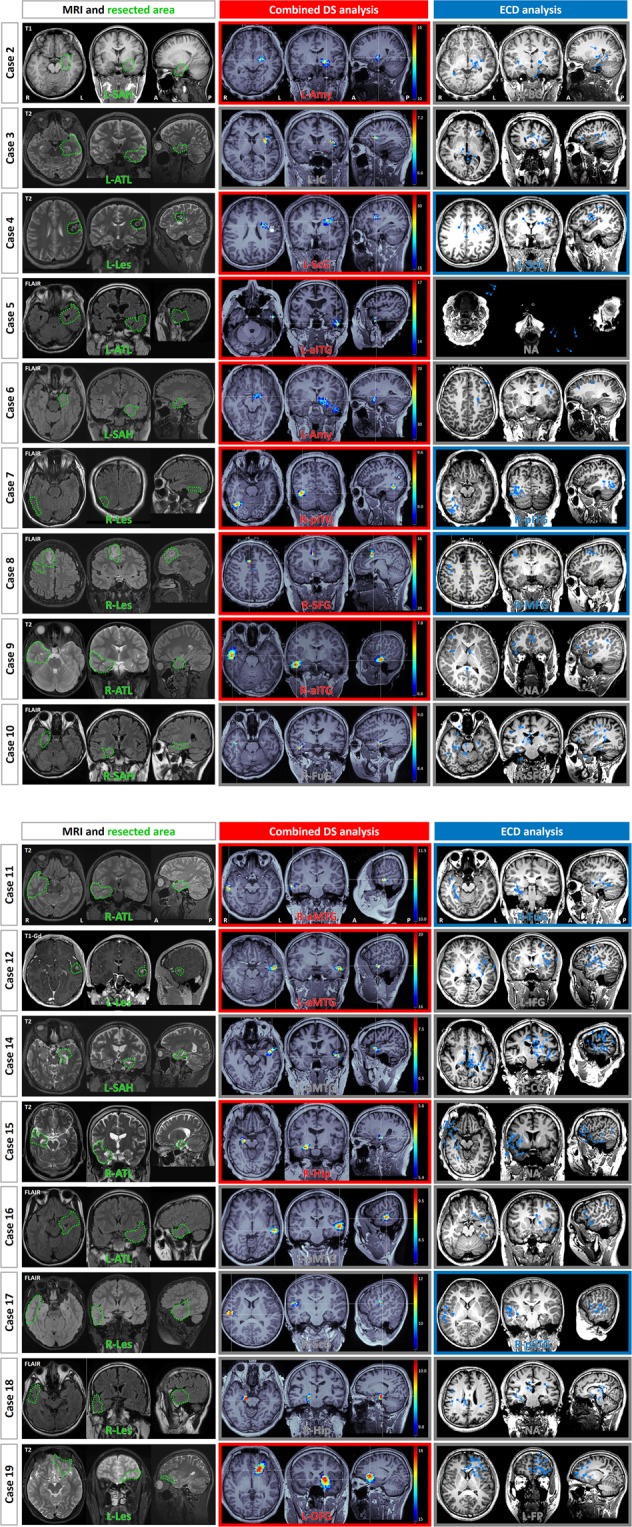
Resected area, cDS analysis, and ECD analysis of 16 cases. Left column: The green dotted line on the preoperative MRI represents the resected area and the surgical method are shown. Middle and right columns: Results of the cDS and ECD analyses showing the diagnosed focus area. When the estimated focus of cDS and ECD analyses was concordant with the resected area, the focus was described by colored letters and a colored frame. When they were discordant, the letters and frame were gray. Abbreviations: cDS, combined distributed source; ECD, equivalent current dipole; SAH, selective amygdalohippocampectomy; ATL, anterior temporal lobectomy; Les, lesionectomy; CC, corpus callosum; Hip, hippocampus; Amy, amygdala; FuG, fusiform gyrus; a-, anterior; p-, posterior; STG, superior temporal gyrus; MTG, middle temporal gyrus; ITG, inferior temporal gyrus; FP, frontal pole; SFG, superior frontal gyrus; MFG, middle frontal gyrus; IFG, inferior frontal gyrus; OFG, orbitofrontal gyrus; ScG, subcentral gyrus; IC, insular cortex; BG, basal ganglia; NA, not available.
